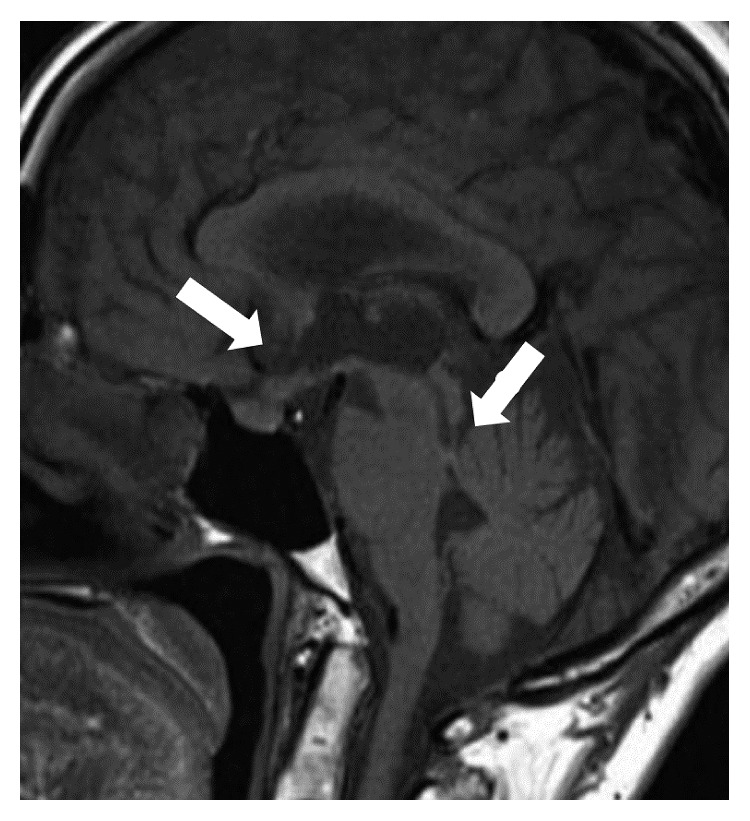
Head MRI showing thickening of the floor of the third ventricle and lesions in the arachnoid and pia membranes (arrows).
